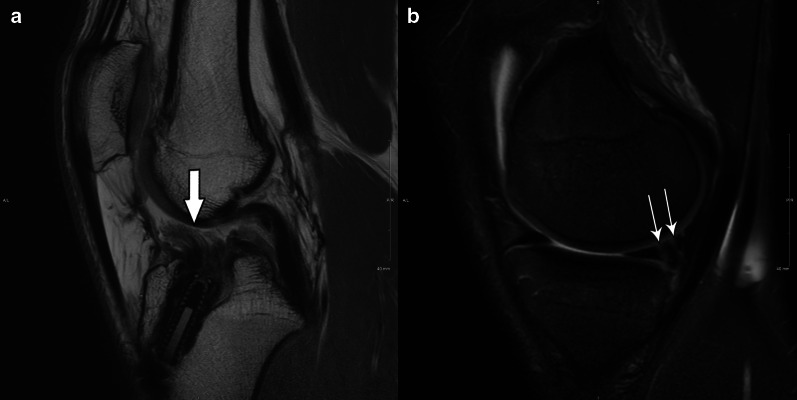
Type 5 meniscal ramp lesion MRI case. Sagittal PD-weighted fat-suppressed MR image (a, left) shows a postoperative ACL graft tear (arrow), and sagittal T2-weighted fat-suppressed (b, right) shows a double vertical peripheral tear through the posterior horn of medial meniscus (thin arrows). *Case courtesy of Dr. Diego Lemos (USA)
